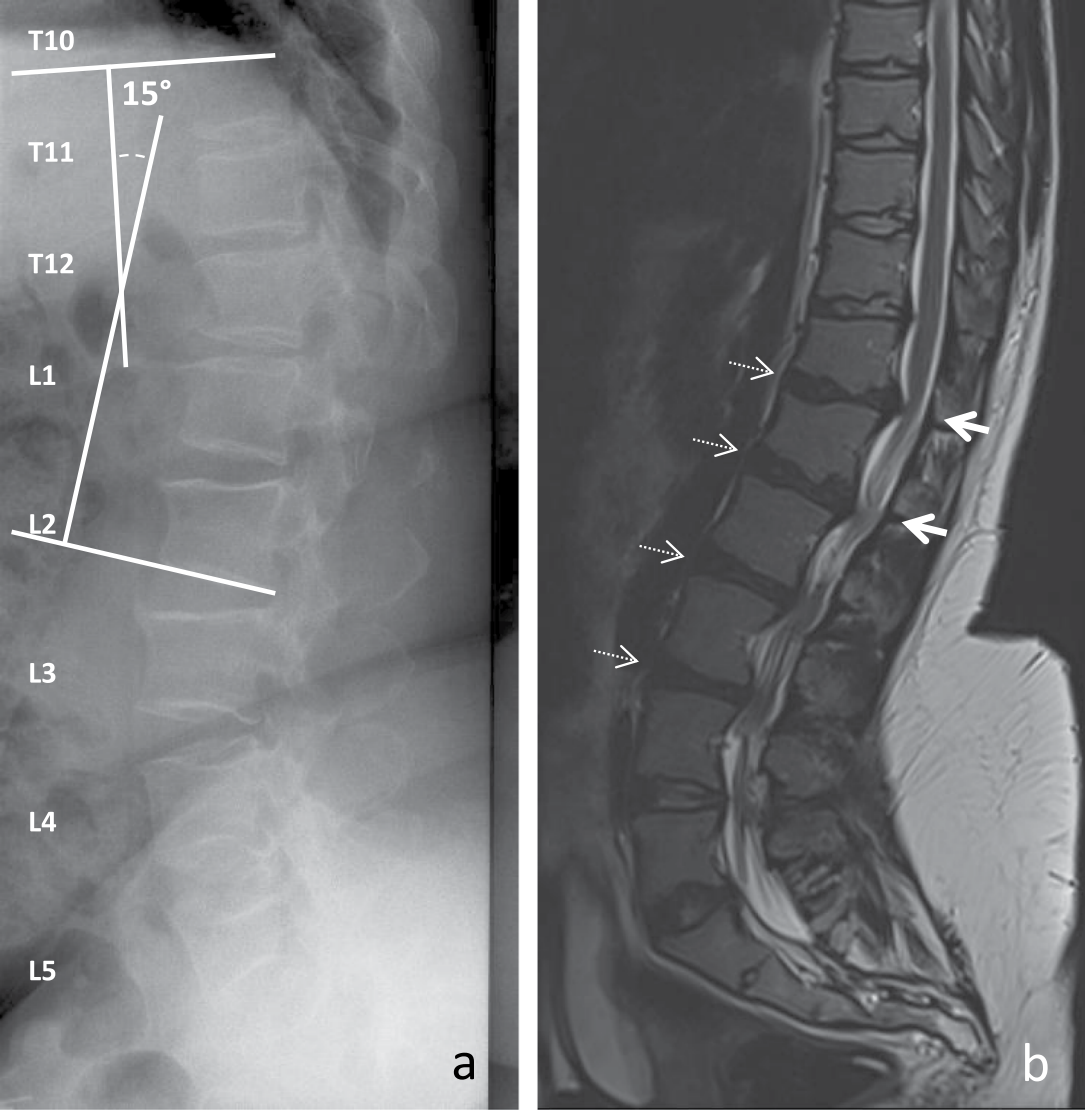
Representative lumbar spine imaging in adults with achondroplasia. (a) X-ray of a 58 year-old women revealing a thoraco-lumbar kyphosis. (b) T2-weighted MRI of a 25 year-old women showing an involvement of the upper part of the lumbar spine including T12-L2 antero-posterior stenoses (thick arrows) and T12-L4 disc degeneration (dark discs, dashed arrows).
